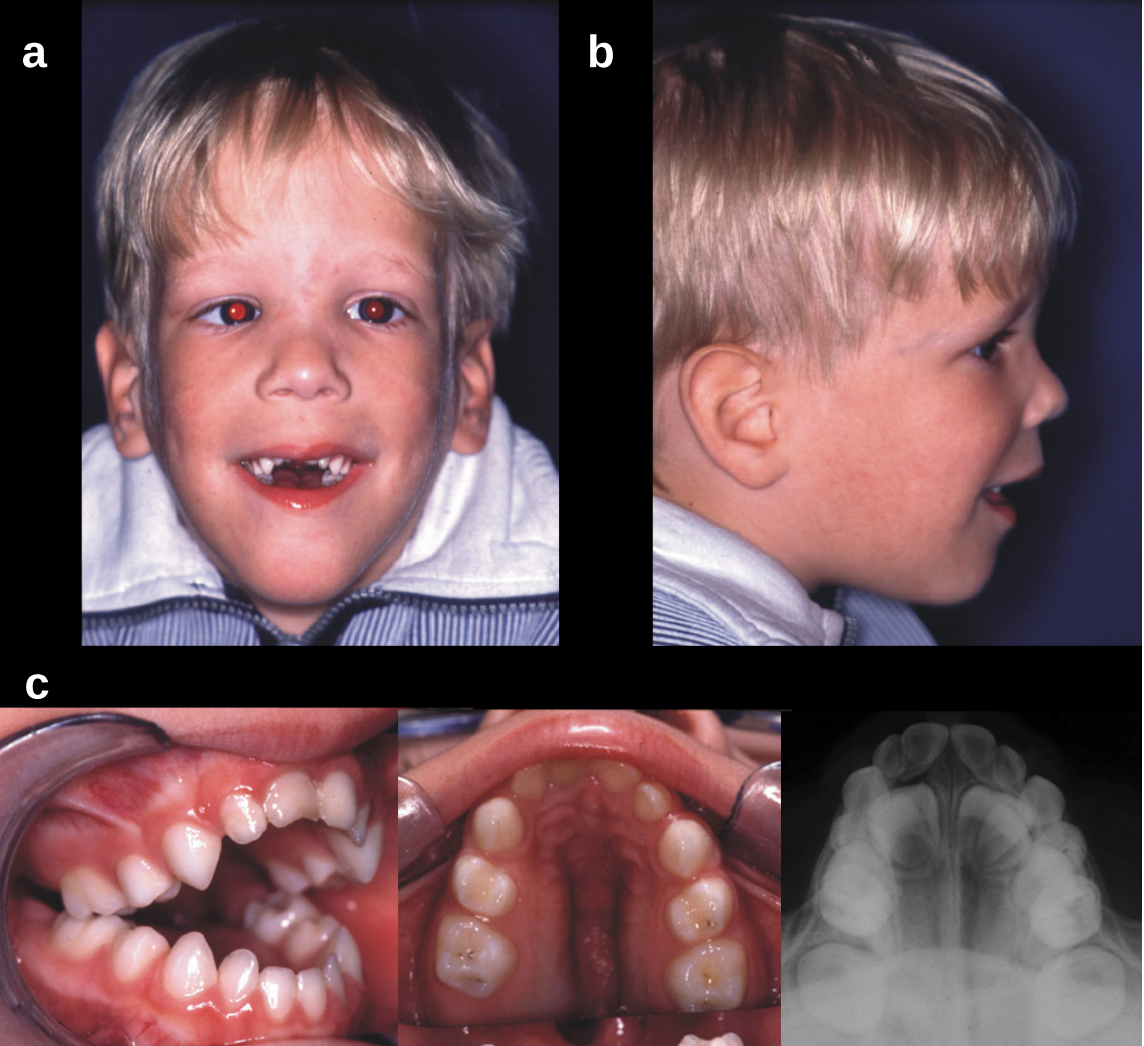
a-e Facial appearance (a, b), circular open bite (c) and palatal aspect (d) of a postnatally orotracheally intubated preterm infant in the initial phase of the dentition. Notice that the teeth of the child are '*in occlusion*' on Figures a-c. The food intake – limited to soft or mashed foods due to the extreme dysgnathia – leads to marked frustration. The infant is teased because of its eating problems and the shape of its jaw and head. Radiography revealed premature ossification of the median suture (e).
